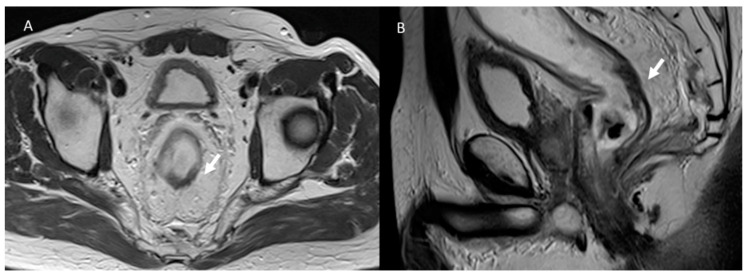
T2-W MRI assessment in post-treatment rectal cancer in the axial (A) and sagittal (B) plane. Arrows show fibrotic changes of the rectal wall without a residual lesion.
